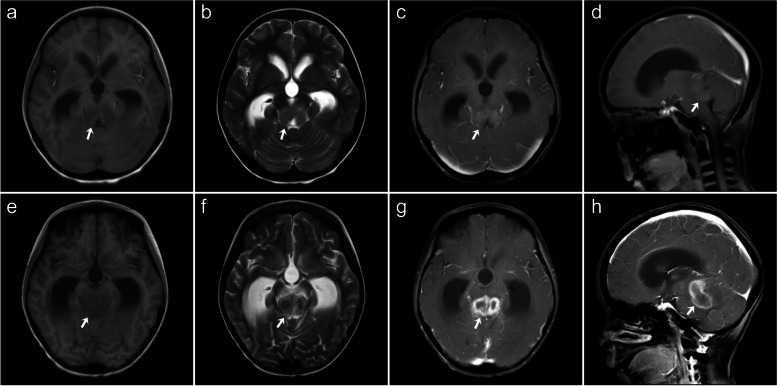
Brain MRI findings for case 3. Brain MRI images (a–d) at the time of initial diagnosis showing a homogenous lesion (white arrows) being hypointense on T1-weighted imaging (a), isointense on T2-weighted imaging (b), with mild homogeneous enhancement (c, d), MRI images (e–h) obtained 18 days after an initial presentation showing the lesion (white arrows) being enlarged in size (e), more heterogeneous in appearance (f), and having a new rim-enhancing feature (g, h)
